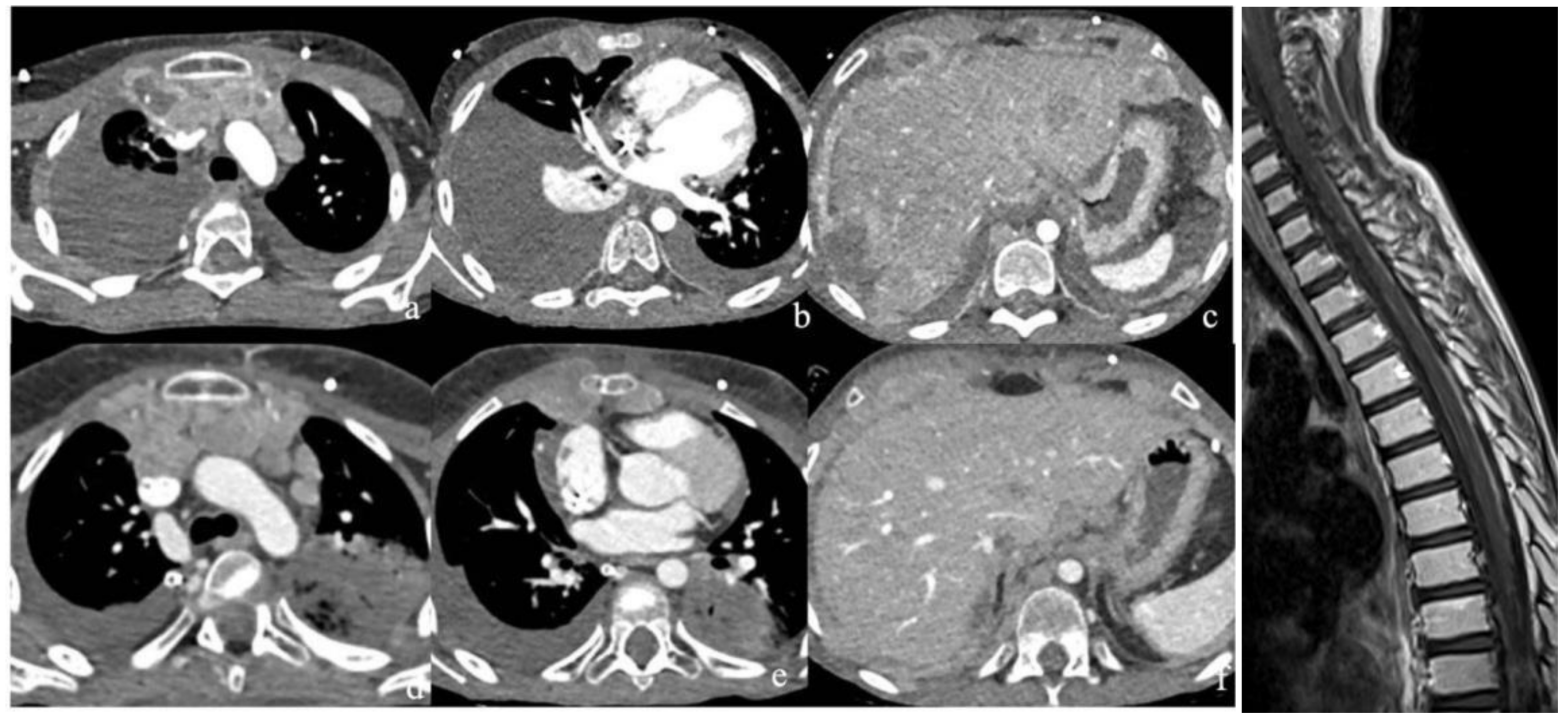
(a–c) CT obtained soon after the clinical deterioration of the patient (upper row) documented disease progression in the chest and abdomen due to the appearance of multiple lymphadenopathies and pleural and peritoneal nodules with corresponding effusion. There was concurrent CNS progression at MR (g); (d–f) Follow-up CT one month later (lower row) showed further disease progression.
